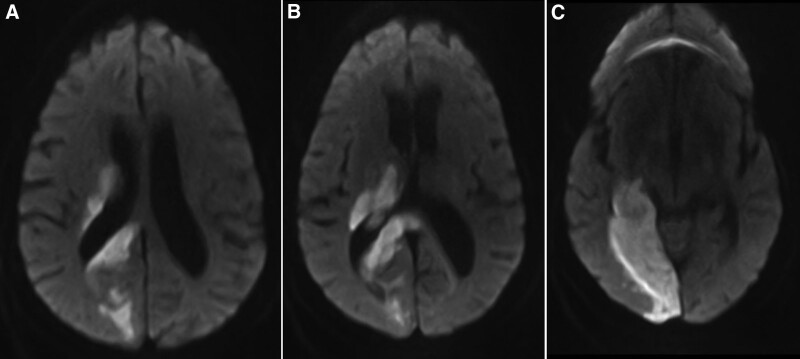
The brain MRI on the day of onset. Diffusion weighted imaging (DWI) scan showed hyperintensity shadow in the right paraventricular, corpus callosum and temporo-occipital lobe.
